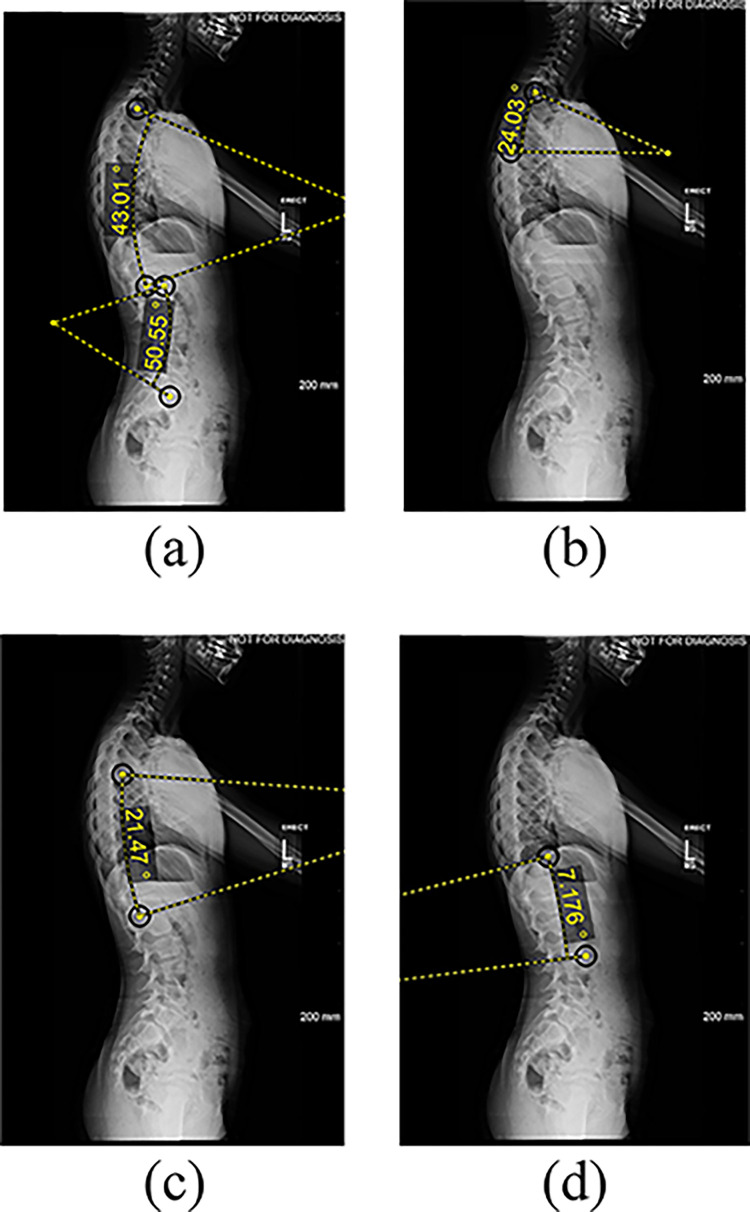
Features extracted from lateral radiographs. (a) thoracic kyphosis and lumbar lordosis (b) proximal thoracic kyphosis (c) mid lower thoracic kyphosis (d) thoracolumbar lordosis angle measured between the superior endplate and the inferior endplate of the vertebral levels: T2-T12, L1-L5, T2-T5, T5-T12, and T12-L2, respectively.
